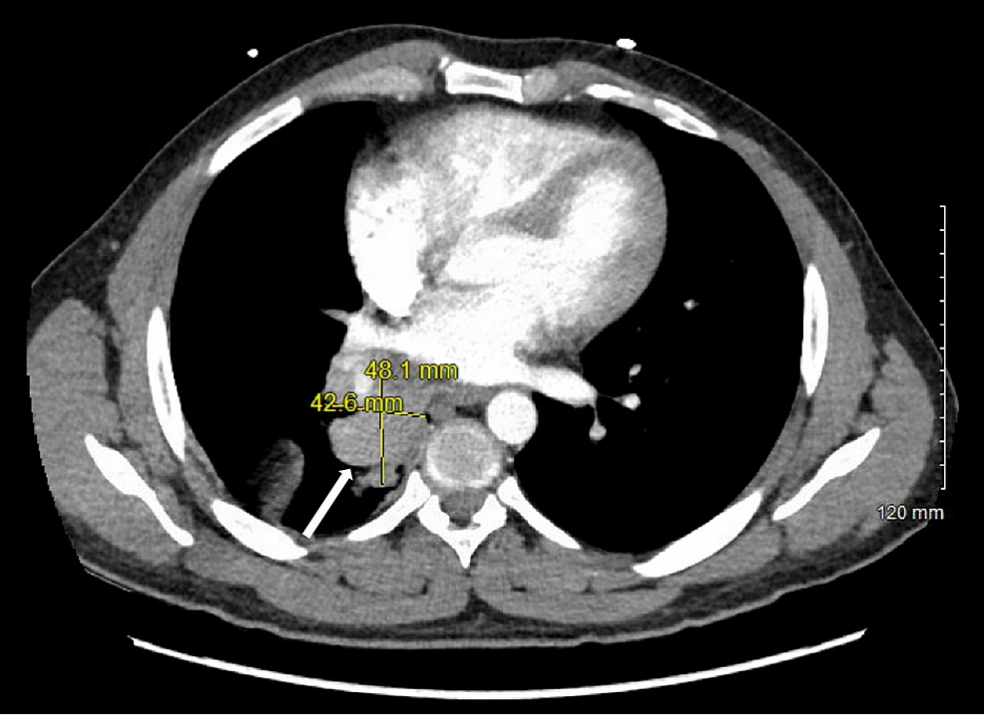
Axial view of computed tomography angiography of the chest with contrast per the pulmonary embolism protocol, demonstrating persistent soft tissue density at the right hilum indicative of an obstruction (42.6mm×48.1mm)
